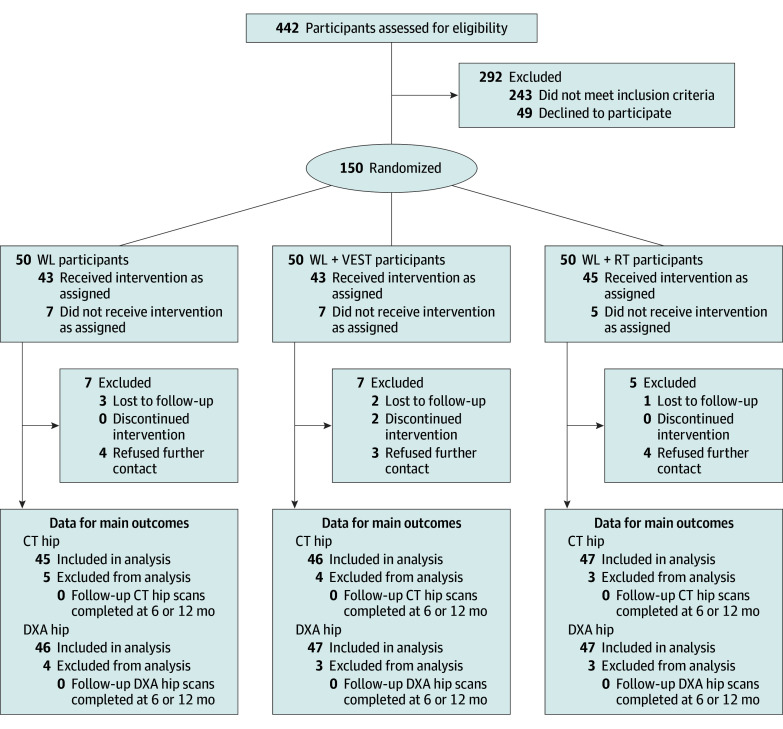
Participant Flow Through the Incorporating Nutrition, Vests, Education, and Strength Training (INVEST) in Bone Health Trial CT indicates computed tomography; DXA, dual-energy X-ray absorptiometry; WL, weight loss; WL+RT, weight loss plus resistance training; WL+VEST, weight loss plus weighted vest.
